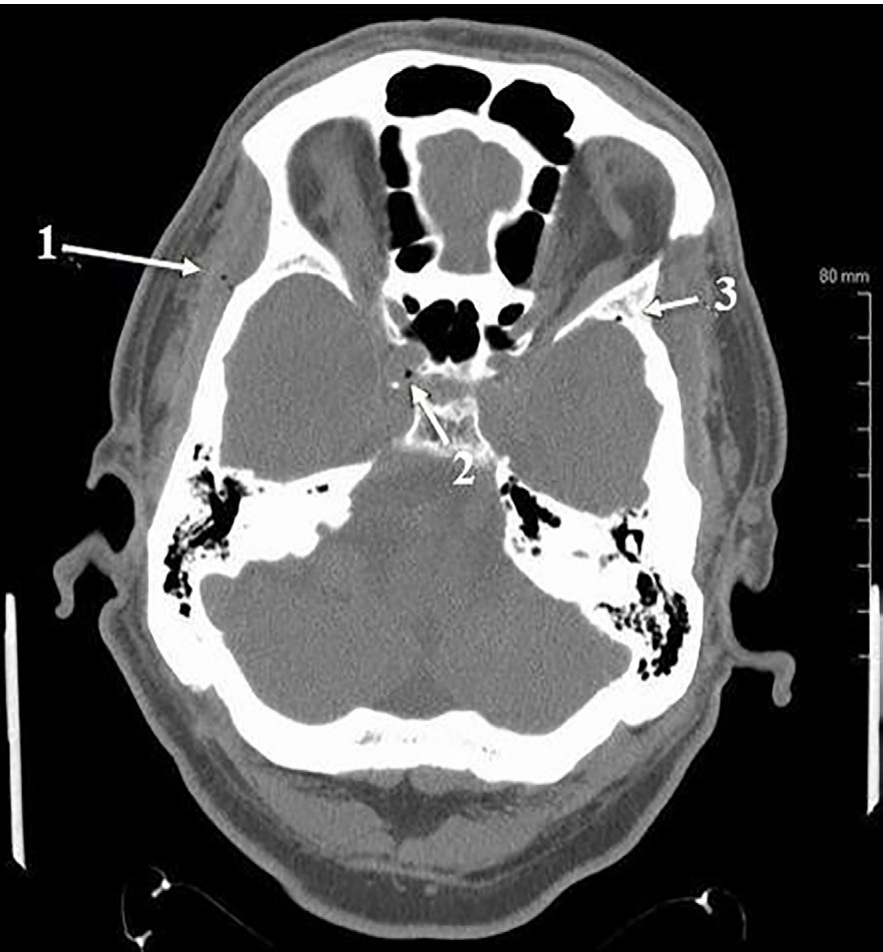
Intracranial air noted on patient’s computed tomography of the head. Arrow 1 indicates multiple foci of air within the right temporalis muscle. Arrow 2 indicates a focus of gas within the cavernous sinus, part of the intracranial venous drainage system. Arrow 3 indicates focus of gas within the sphenoparietal sinus, also part of the intracranial venous drainage system.
